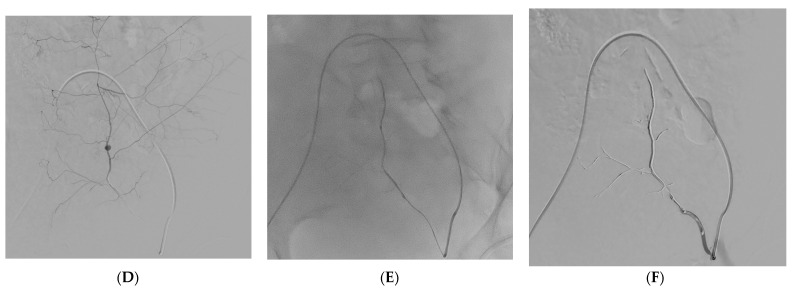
A 57-year-old man with coagulopathy, COVID-19, abdominal pain, and acute anaemia. (A–C) CT angiography demonstrating a rectus sheath hematoma with a pseudoaneurysm of the left inferior epigastric artery depicted in the oblique sagittal and coronal planes by maximum intensity projection reformatting. (D) Digital Subtraction Angiography showing selective catheterization and pseudoaneurysm of the inferior epigastric artery. (E,F) Fluoroscopy showing the Onyx 34 cast distributed along the inferior epigastric artery and Digital Subtraction Angiography confirming successful embolization.
